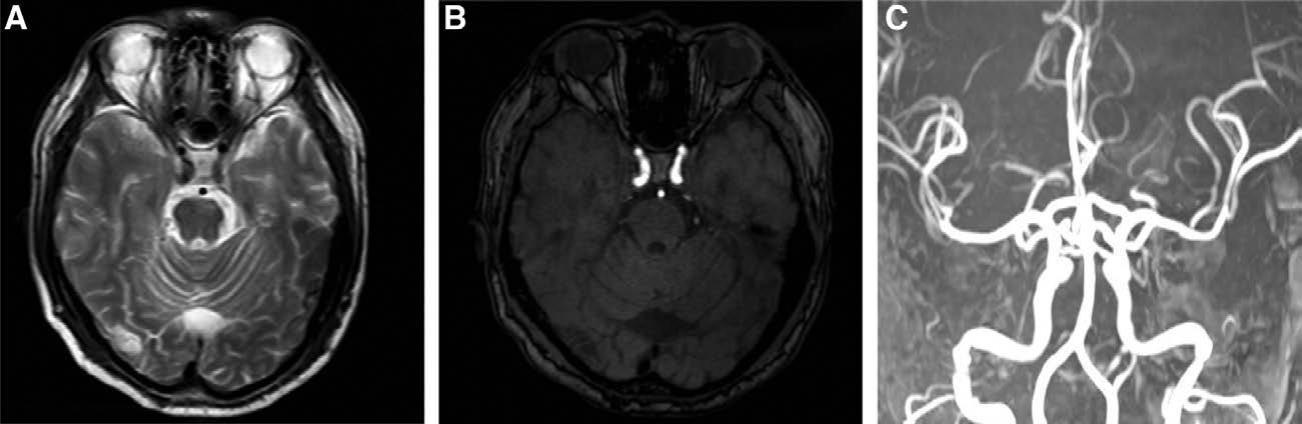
MRI (A) and MRA (B and C) examination with no positive results. MRA = magnetic resonance angiography, MRI = magnetic resource imaging.
